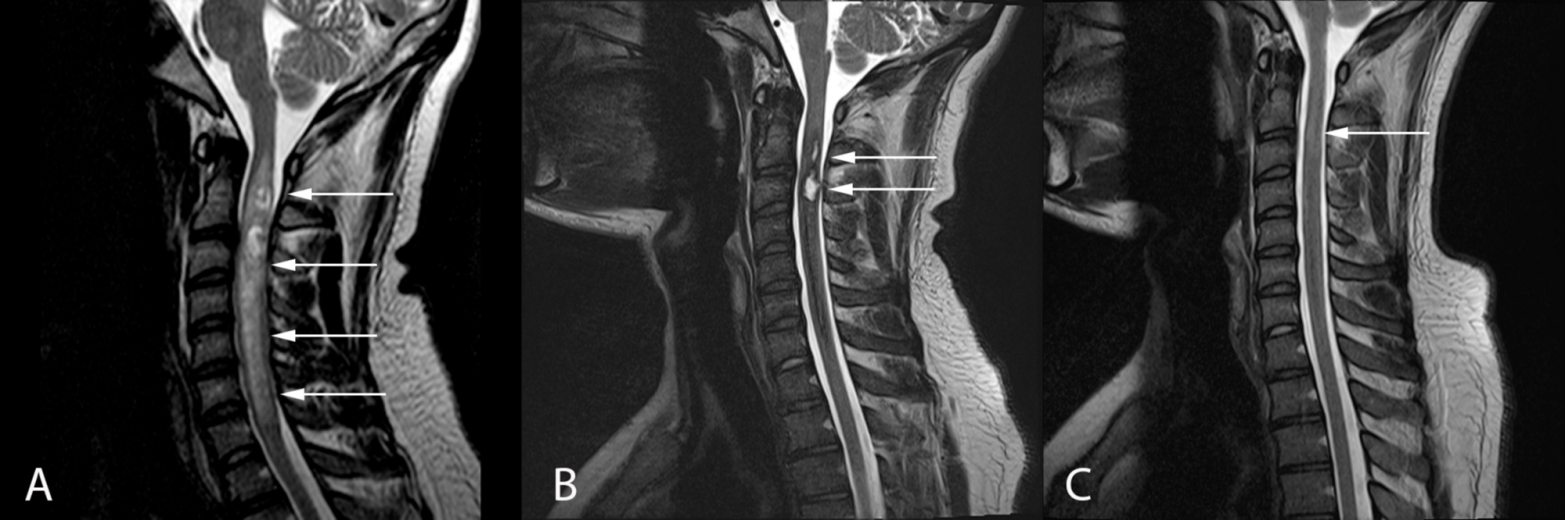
Sagittal T2-weighted MRI at patient presentation (A), three-week follow-up (B), and nine-week follow-up (C) depicting almost complete spontaneous resolution of the cervical intramedullary spinal cord lesion (arrows)
